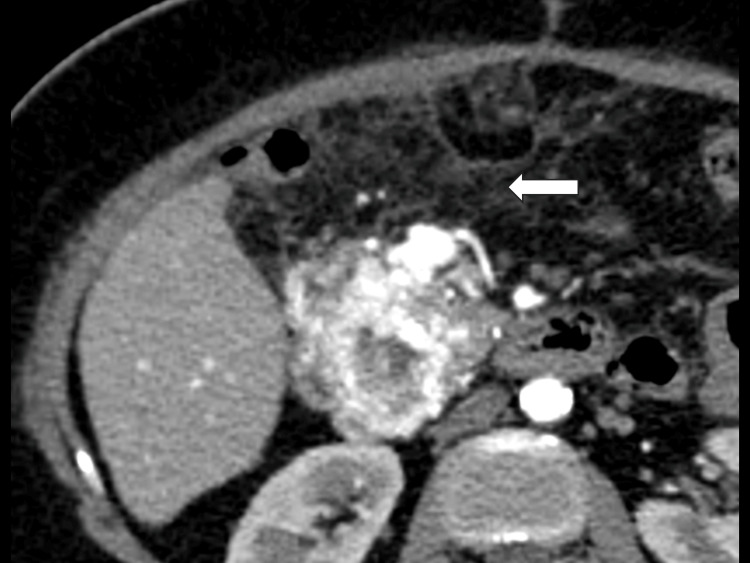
Follow-up imaging showing inflammatory changes Contrast-enhanced CT scan of the abdomen, axial plane in the early postoperative period after exploratory laparotomy and incisional biopsy of the pancreatic mass, again demonstrating a heterogeneously hyperenhancing lesion in the pancreatic head without significant change in size, with increased attenuation and stranding of the surrounding peripancreatic and mesenteric fat (arrow), findings compatible with inflammatory and postsurgical changes. CT: computed tomography
